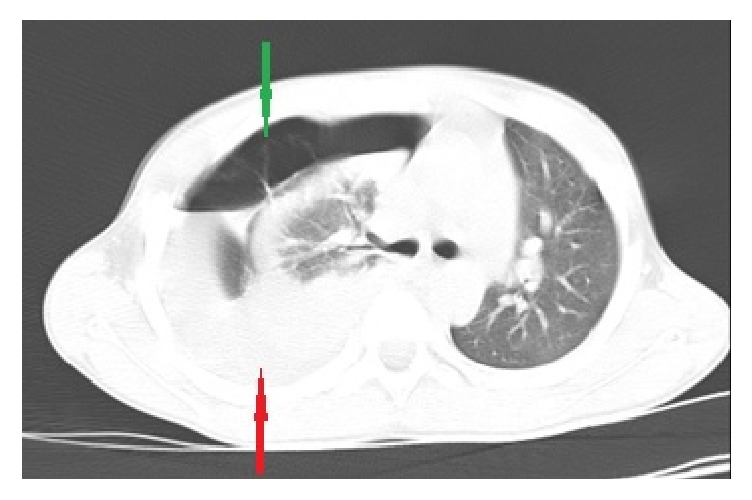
Thoracic CT scan showing the presence of digestive loops (green arrow), and right lobe of the liver (red arrow) with compression of the right lung and shift of the mediastinum to the left.
